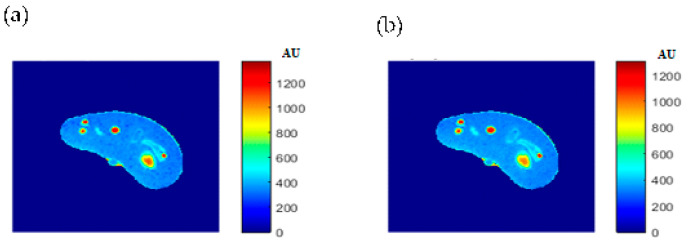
(a) The real MR image, and (b) the generated MR image using *T_E_* and *T_R_* of 130 ms and 2000 ms, respectively.
